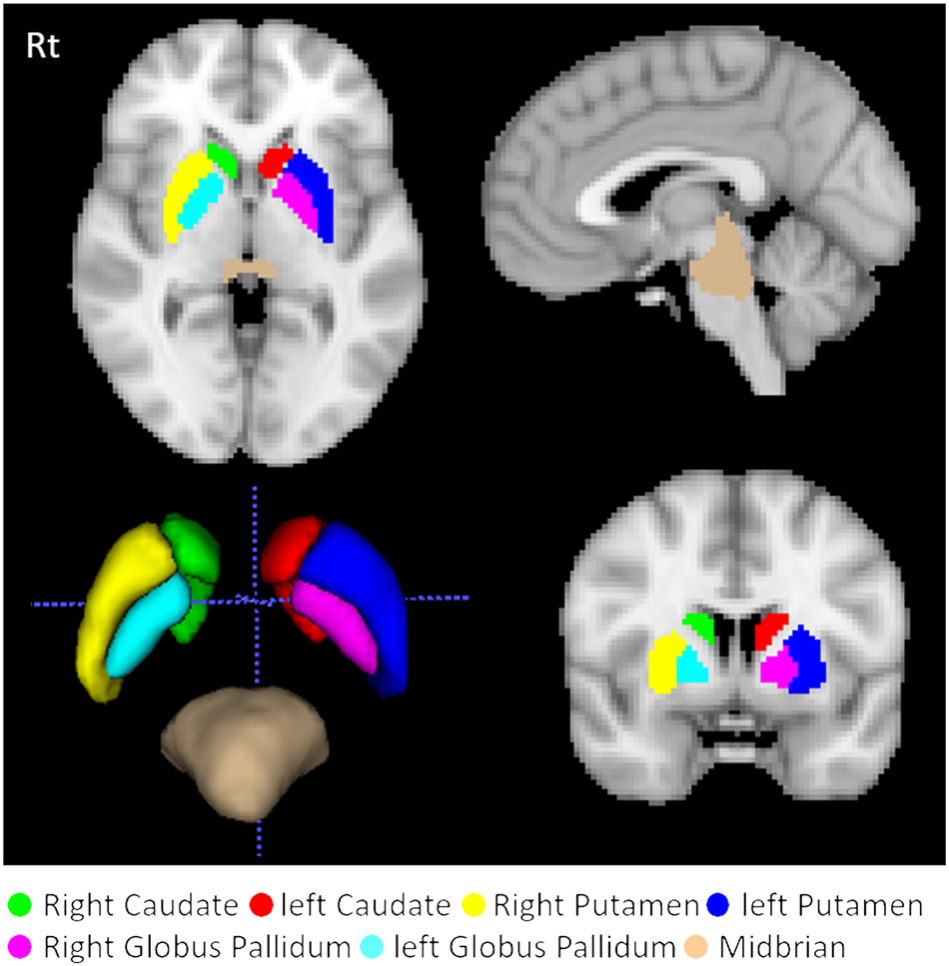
VOIs for the DAT-SPECT analysis.
